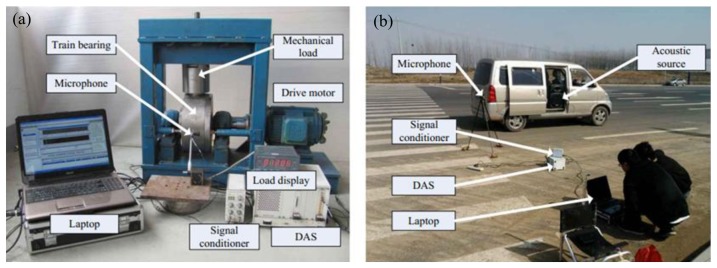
Experimental setup for acoustic signal acquisition with the Doppler effect: (a) test bench of the first experiment and (b) actual scene of the second experiment.
